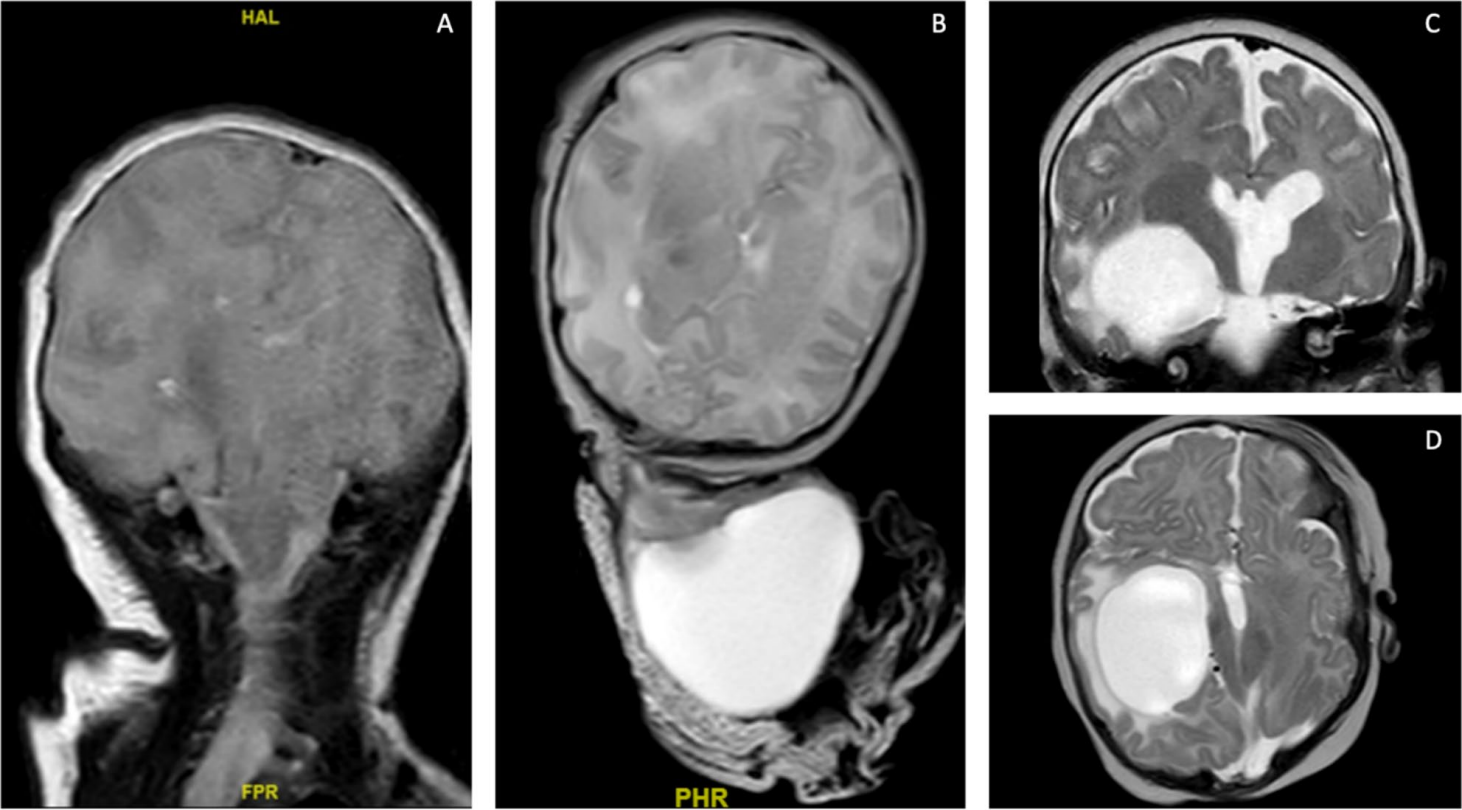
Postnatal, pre-surgical T2-weighted coronal (A) and T2-weighted axial (B) MRI: right temporal arachnoid cyst nonvisible. Post-operative control MRI 9 months after the surgical correction of the encephalocele, T2-weighted coronal (C), and T2-weighted axial MRI (D): development of ventriculomegaly and a right temporal arachnoid cyst with mass effect and deviation of the midline structures
